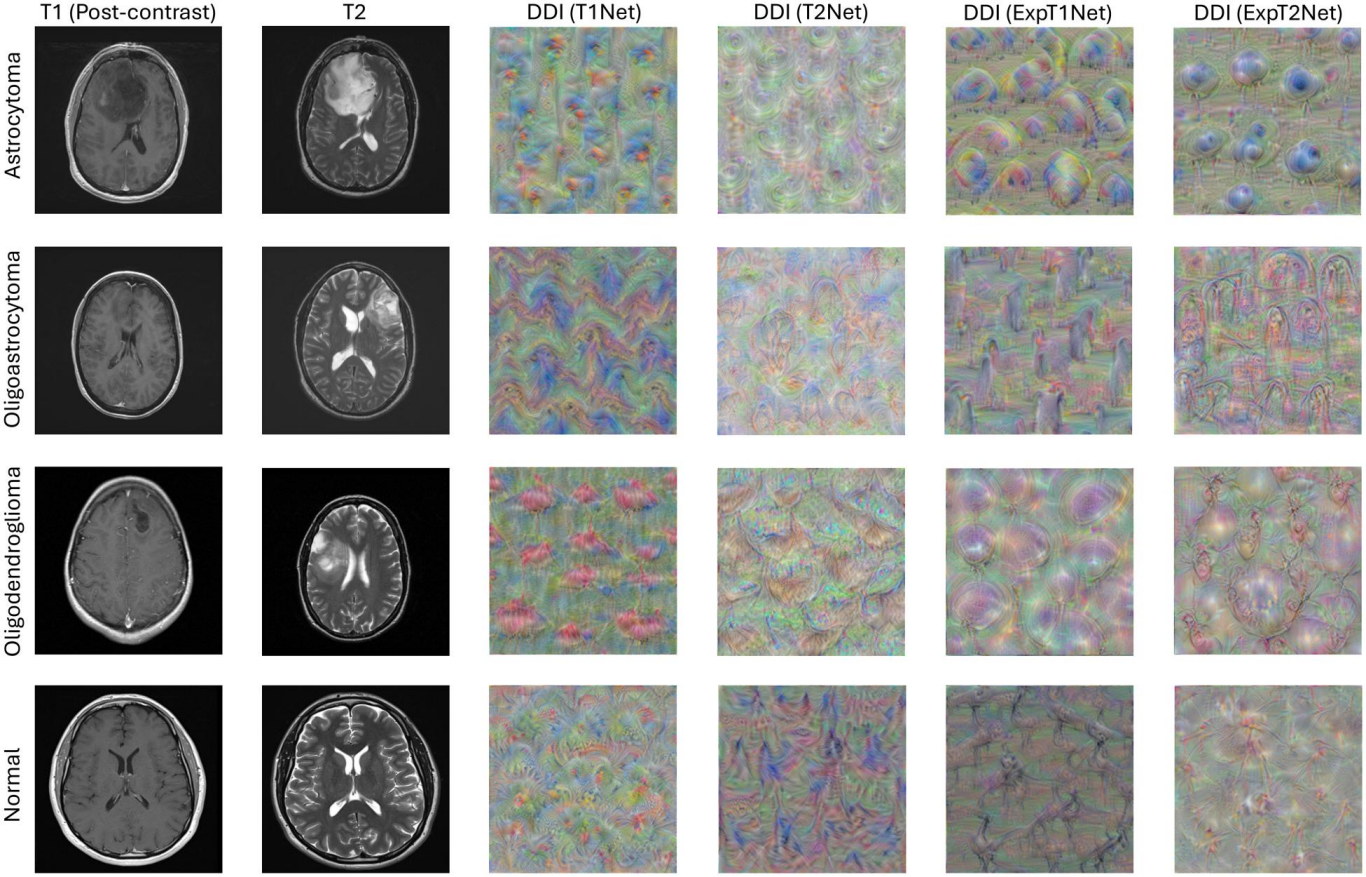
DeepDreamImage (DDI) images of each category of tumor (rows) generated by each trained network (columns 3–6). Sample post-contrast T1-weighted (column 1) and T2-weighted (column 2) MRIs are shown for visual comparison of tumor characteristics (*feature prints)* to those generated through DDI. Feature prints appear to have more distinct forms and shapes for all tumors relative to control (rows 1–3), with transfer learning further enhancing and sharpening the shape of feature prints (columns 5–6)
